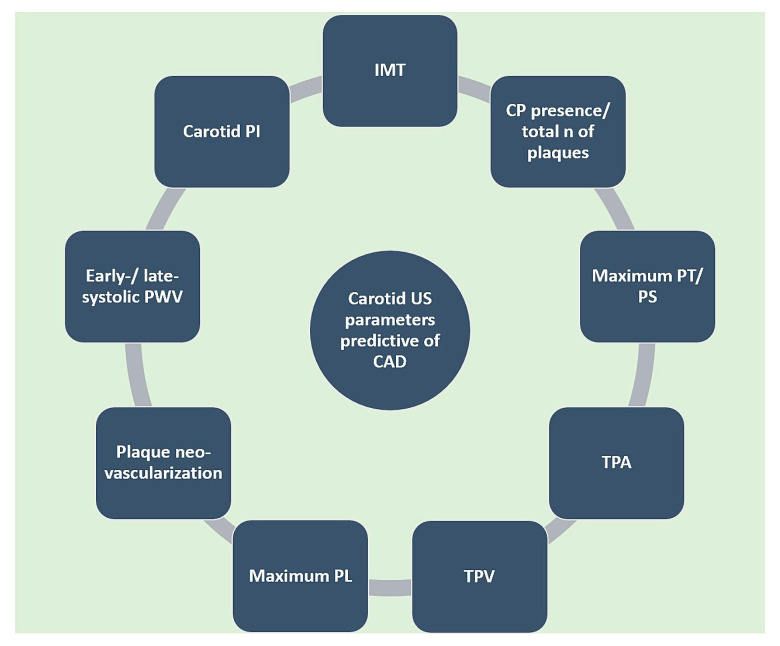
Carotid US-derived parameters predictive of CAD. CAD: coronary artery disease. CP: carotid plaque. IMT: intima-media thickness. N: number. PI: pulsatility index. PL: plaque length. PS: plaque score. PT: plaque thickness. PWV: pulse wave velocity. TPA: total plaque area. TPV: total plaque volume. US: ultrasound.
